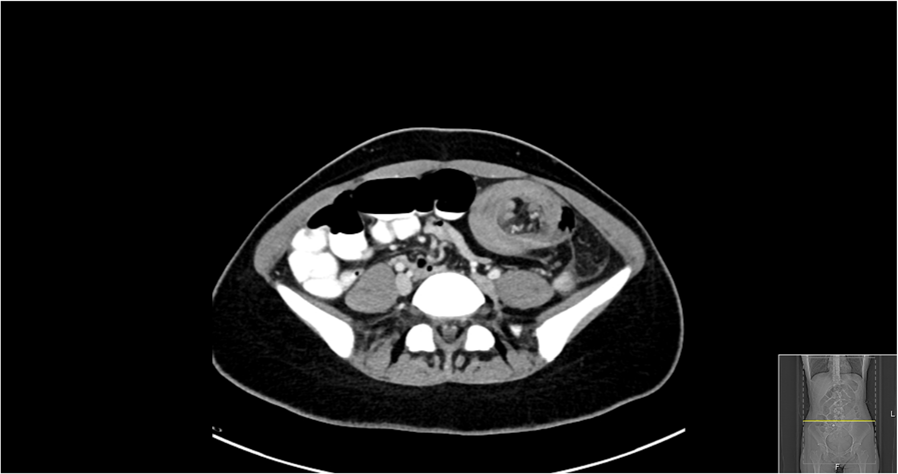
Axial section of abdominal CT revealing "target" sign of ileocolic intussusception in left abdomen.
